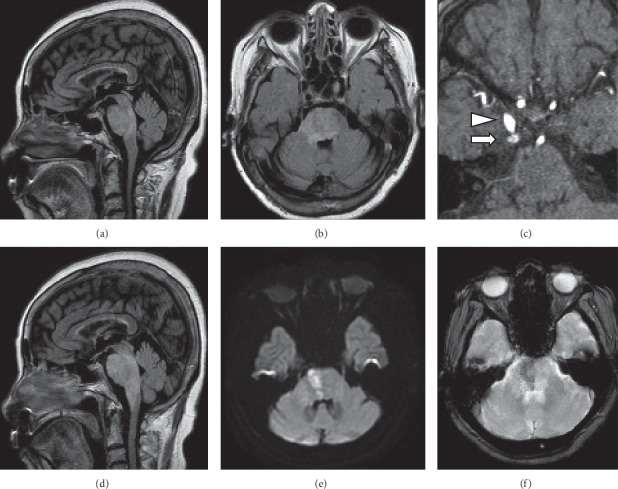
Magnetic resonance imaging. (a, b) Fluid-attenuated inversion recovery (FLAIR) imaging showing widespread hyperintensity diffusely involving the right pons on day 1. (c) Magnetic resonance angiography showing an abnormal flow signal (arrow) posterolateral to the cavernous sinus. Arrowhead indicates the right internal carotid artery. (d) FLAIR imaging. Brainstem edema has worsened and expanded rostrocaudally on day 2. (e) Diffusion-weighted showing shows hyperintense lesion within the edematous pons. (f) T2 star-weighted imaging showing an area of low intensity, suggesting venous congestion and hemorrhage.
